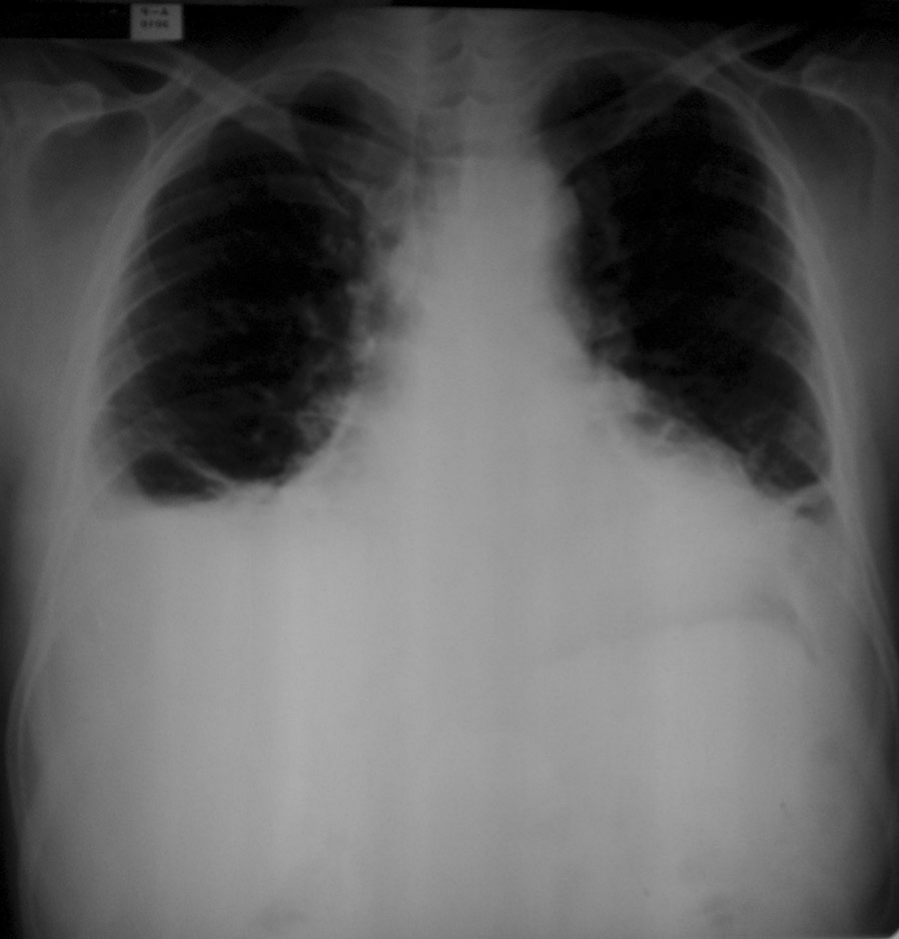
Chest radiograph showing cardiomegaly and bilateral pleural effusions in the lung bases. The chest radiographs reveal bilateral pleural effusion in the lung base and an enlarged cardiac silhouette
